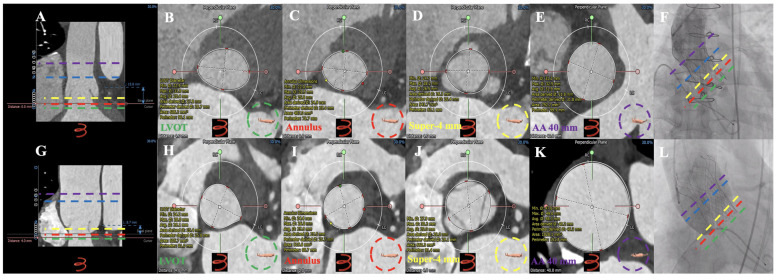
MDCT measurements and successful TAVR in type 2 and type 3 patients with chronic severe AR. CT anatomical classifications—Type 2: (A): longitudinal section of the aortic root; (B): LVOT; (C): annulus; (D): super-annular 4 mm; (E): AA 40 mm; (F): 27 mm Vitaflow valve successfully implanted. CT anatomical classifications—Type 3: (G): longitudinal section of the aortic root; (H): LVOT; (I): annulus; (J): 4 mm above the annulus; (K): AA 40 mm; (L): 30 mm Vitaflow valve successfully implanted. ▬ ▬ ▬ ▬ = Annulus; ▬ ▬ ▬ ▬ = LVOT; ▬ ▬ ▬ ▬ = super-annular 4 mm; ▬ ▬ ▬ ▬  = AA 40 mm; ▬ ▬ ▬ ▬ = sinotubular junction. AR = aortic regurgitation; TAVR = transcatheter aortic valve replacement; MDCT = multidetector computed tomography; LVOT = left ventricular outflow tract; AA = ascending aorta.
